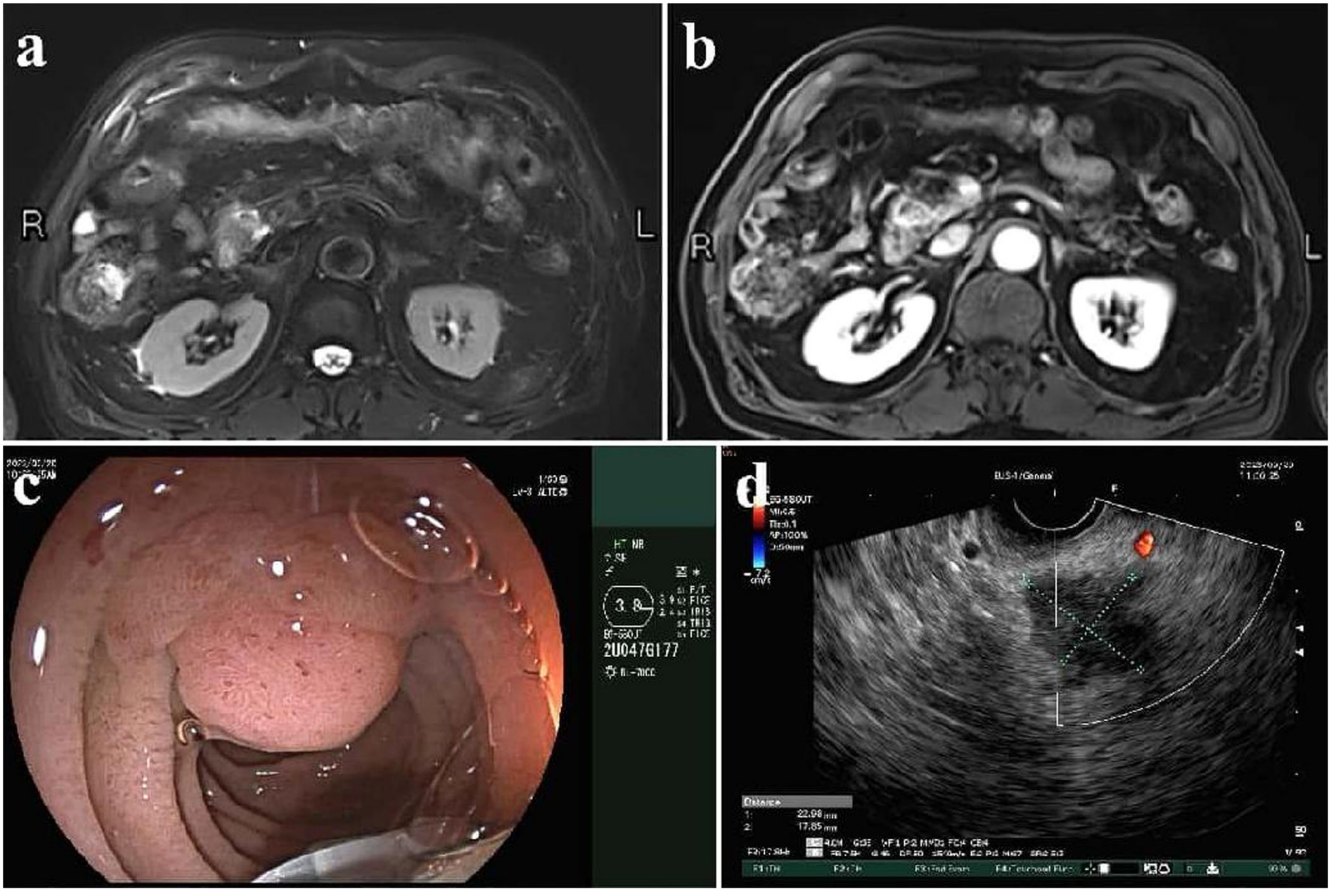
MRI and endoscopic ultrasound showed a mass at pancreatic head. (a) MRI scan; (b) enhanced MRI scan; (c) endoscopic show external compression of the tumor on the duodenal wall; (d) endoscopic ultrasound suggests invasive tumor.
